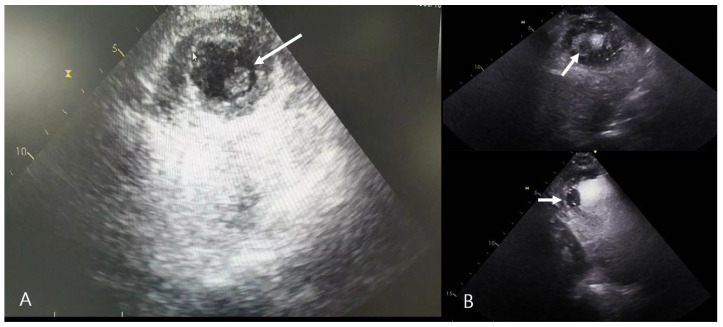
Postoperative echocardiography demonstrates mural thrombi in the left ventricular apex. (A) Transthoracic echocardiography shows an echogenic mass adherent to the left ventricular apex (arrow). (B) Contrast-enhanced echocardiography delineates a non-opacified apical filling defect (arrows), confirming mural thrombosis.
